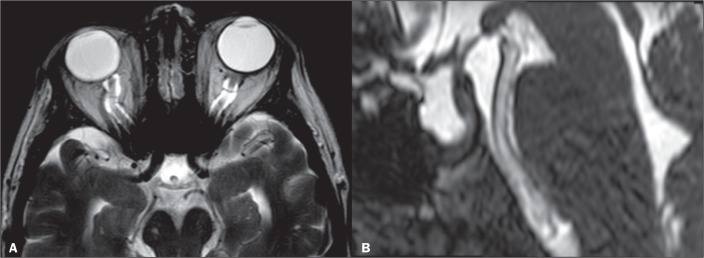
Axial and sagittal T2-weighted MRI scans (A and B, respectively) showing
